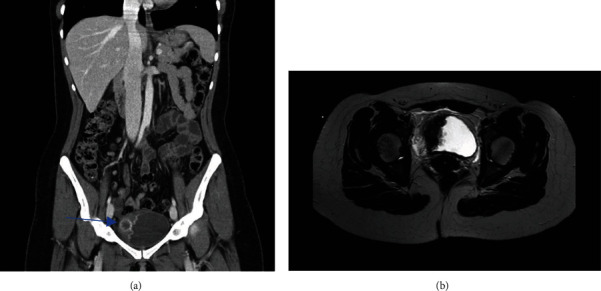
Coronal CT scan (a) and axial MRI (b) demonstrating 2 × 2 cm exophytic polyp (arrow) associated with significant bladder wall thickening of the urinary bladder with invasion of detrusor muscle.
